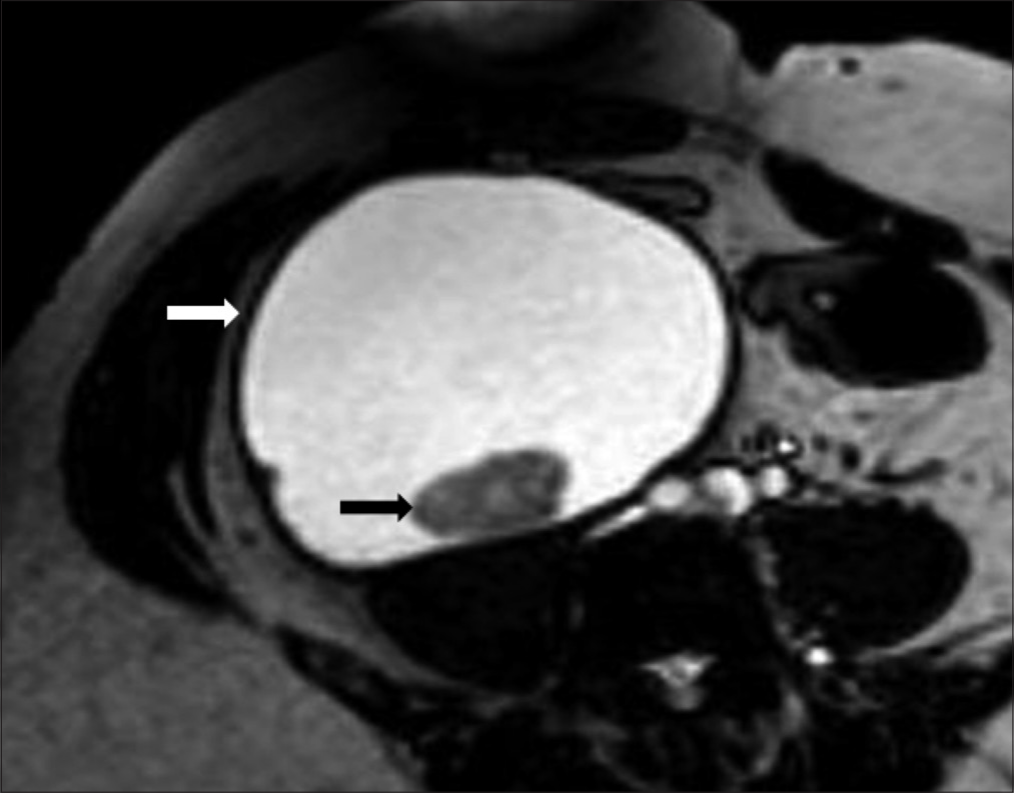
Axial, steady-state, free-precession MRI image shows a hyperintense lesion (white arrow) with a hypointense area within (black arrow). The lesion is predominantly hypointense on T1W and hyperintense on T2W images, indicating a cystic lesion containing debris
